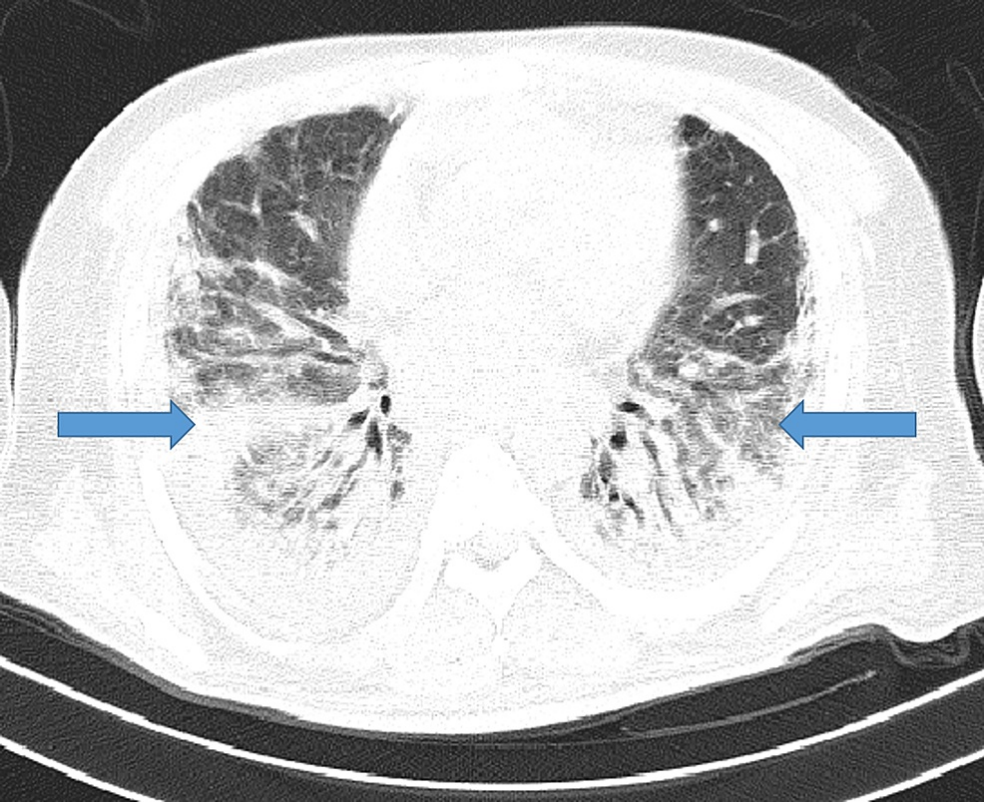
HRCT of the thorax showing bilateral ground-glass opacities and consolidation. HRCT: high-resolution computed tomography
